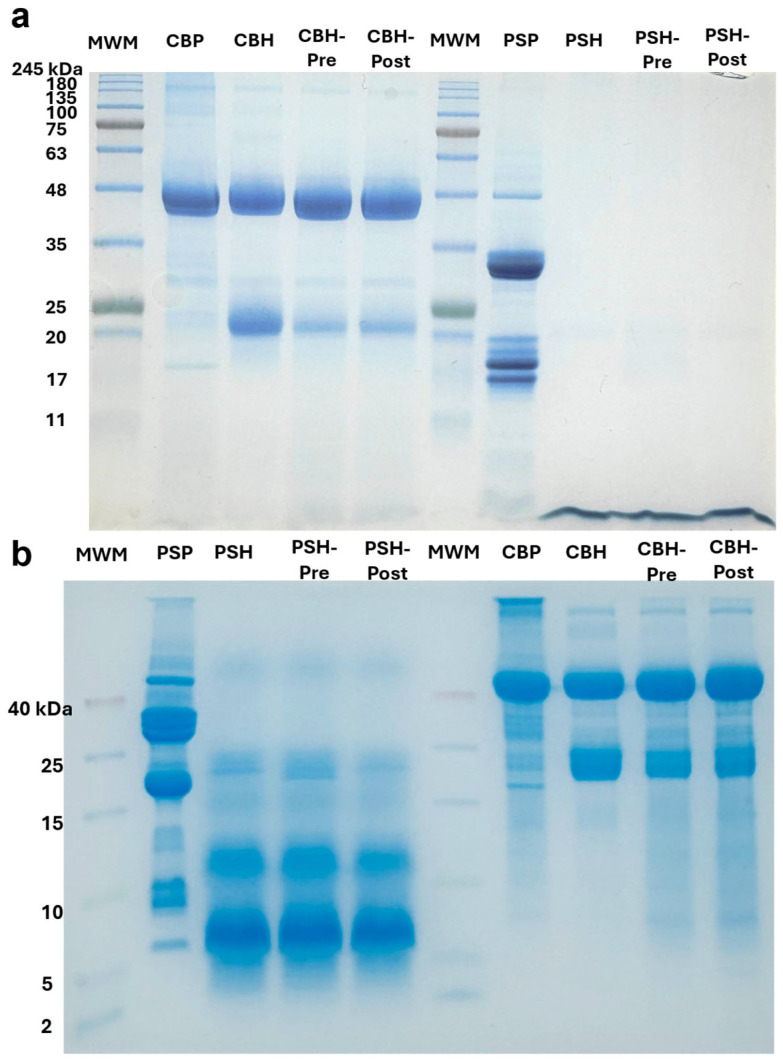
Protein and peptide profiles of common bean and pumpkin seed. (a) SDS-PAGE profile of CBP and PSP and their ultrasound-assisted hydrolysates; (b) Tricine–SDS-PAGE profiles of CBP and PSP and their ultrasound-assisted hydrolysates. MWM: molecular weight ladder, CBP: common bean protein, CBH: common bean hydrolysate, CBH-Pre: ultrasound pre-treatment of CBH, CBH-Post: ultrasound post-treatment of CBH, PSP: common bean protein, PSH: common bean hydrolysate, PSH-Pre: ultrasound pre-treatment of PSH, PSH-Post: ultrasound post-treatment of PSH.
